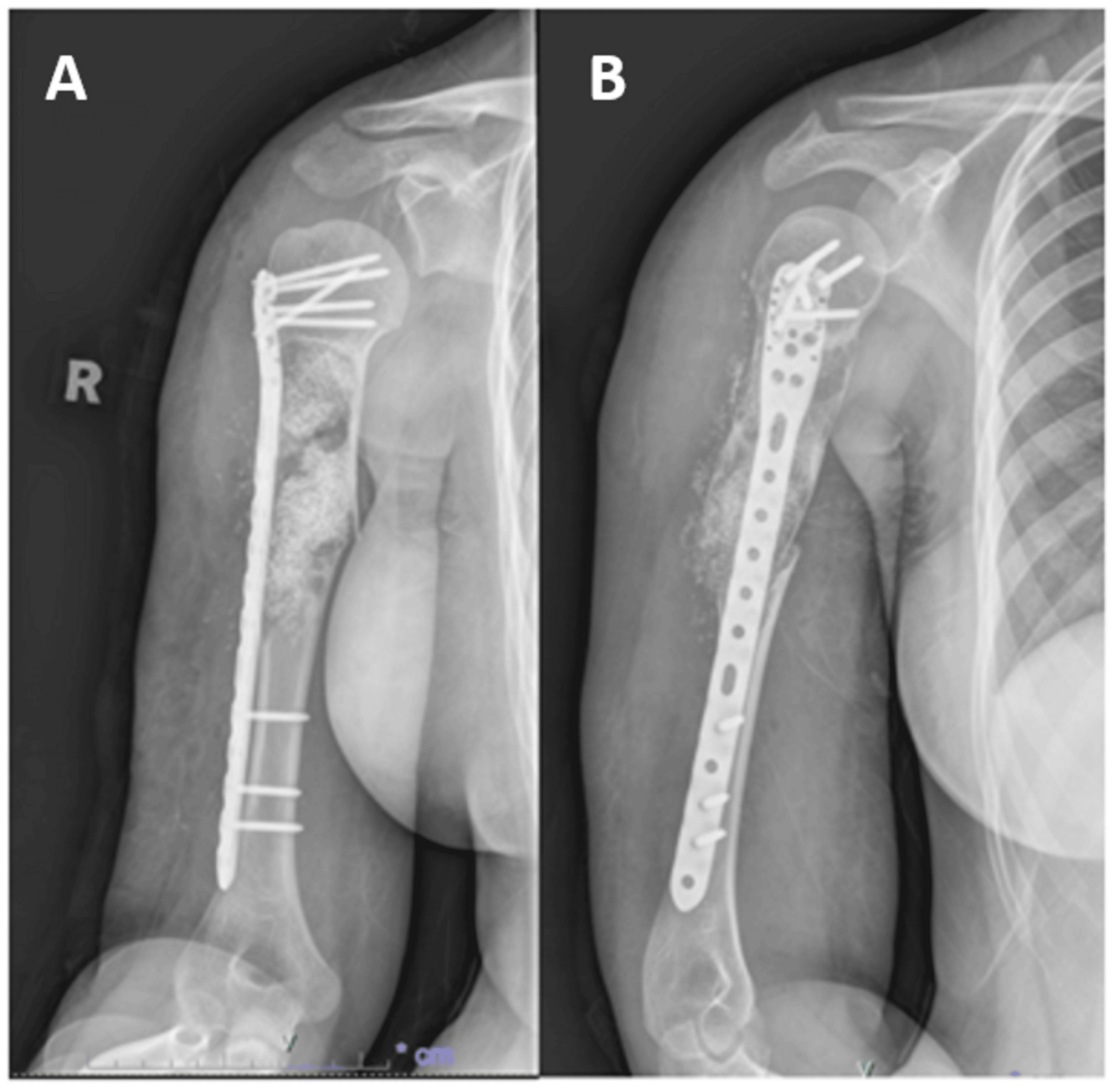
Radiograph of right humerus in anteroposterior and lateral view on postoperative day one A: Anteroposterior view, B: Lateral view
